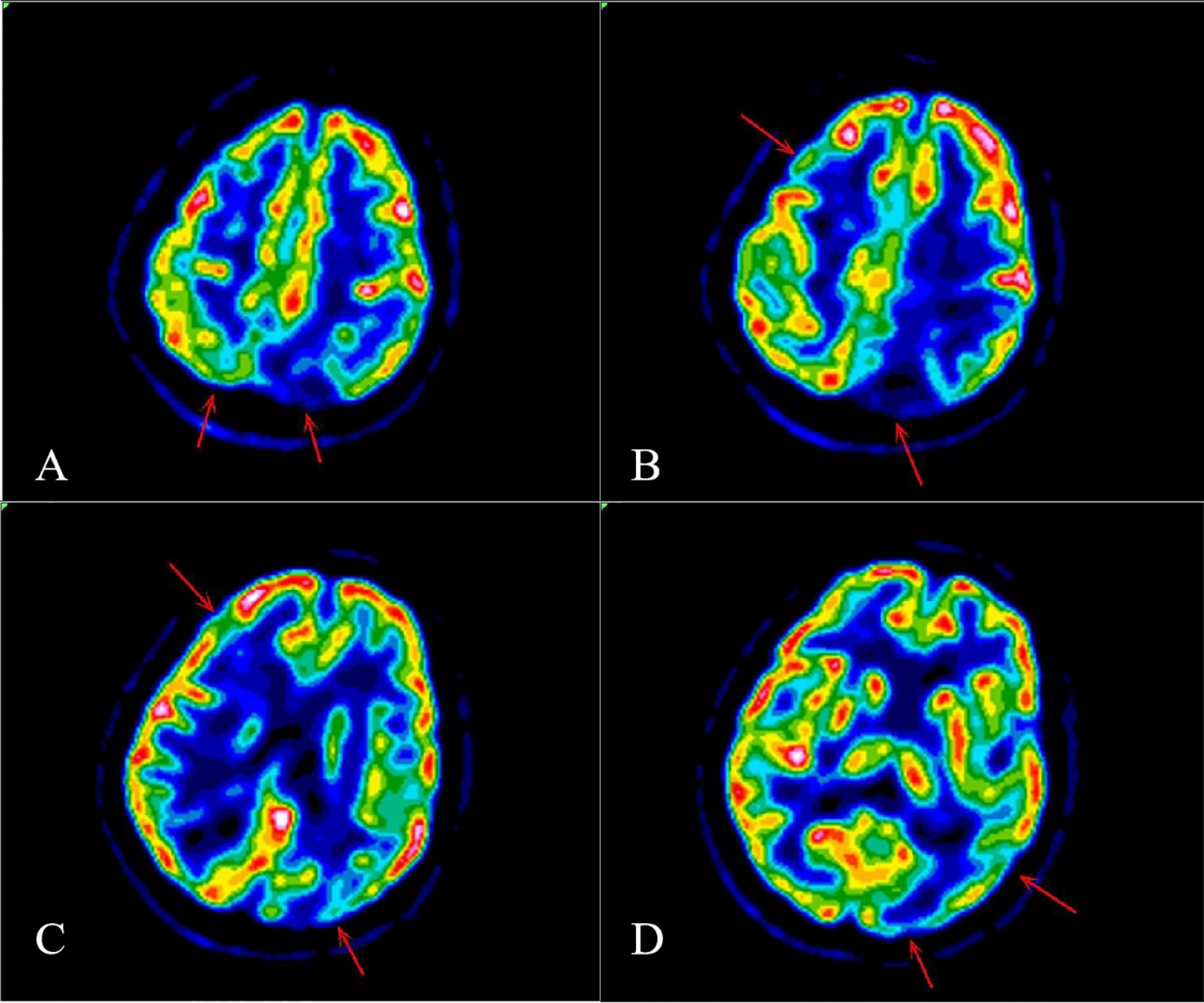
PET-CT showed decreased cortical metabolism in the right frontal lobe, parietal lobe, occipital lobe and left temporal lobe (A-D).
